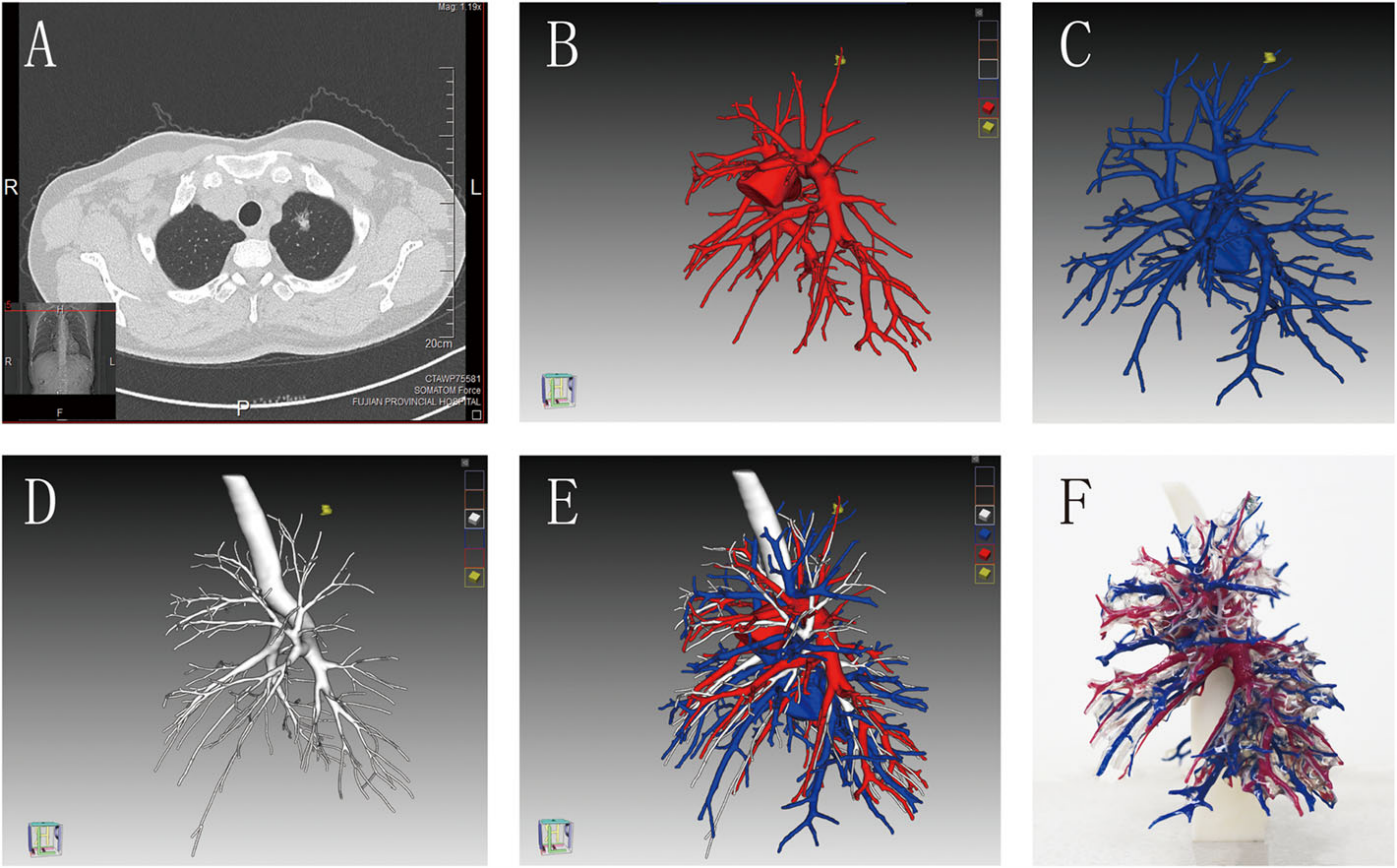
CT image, 3D reconstruction image, and 3D model of the same case. a The patient’s two-dimensional (2D) computed tomography (CT) image showing a part-ground glass nodule (pGGN) in the left upper lung. b–e The patient’s three-dimensional (3D) CT reconstruction image, from which the lung veins, bronchus, and arteries can be more intuitive. f The patient’s 3D print of the lung model not only allows the visualisation of the lung anatomy but also facilitates coordinate positioning, accurately locates the lesion, and helps in the precise wedge resection of the lesion during surgery
